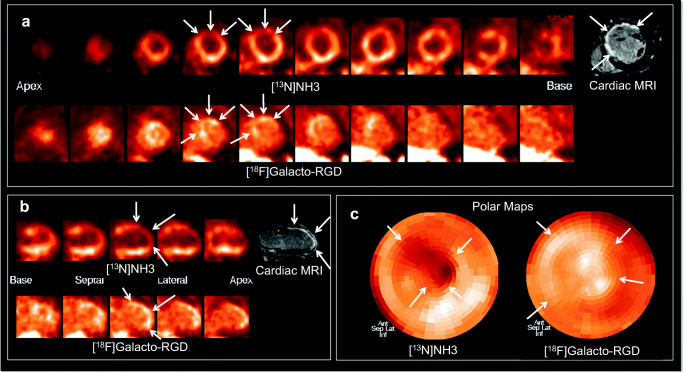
Patterns of [^18^F]Galacto-RGD uptake in myocardial infarction. [^13^N]NH_3_ and [^18^F]Galacto-RGD PET as well as late enhancement cardiac MRI is shown (A: short axis; B: vertical long axis; C: polar maps). A large area of infarction with predominantly transmural contrast enhancement is shown in cardiac MRI in the anterior and anteroseptal wall with severely impaired blood flow in [^13^N]NH_3_ PET (arrows). [^18^F]Galacto-RGD PET shows tracer uptake in the area of infarction; however, note that the tracer uptake also extends to areas adjacent to the infarcted areas into myocardium with normal or only slightly impaired blood flow (arrows), which is especially well demonstrated in the polar maps (C)
